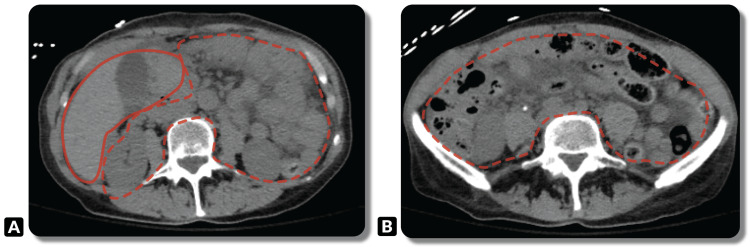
Axial CT angiogram images at two different levels. (A) Portal edema (solid red line) with diffuse mesenteric stranding and edema (dashed red line). (B) Diffuse mesenteric stranding and edema (dashed red line) at a different axial level.
